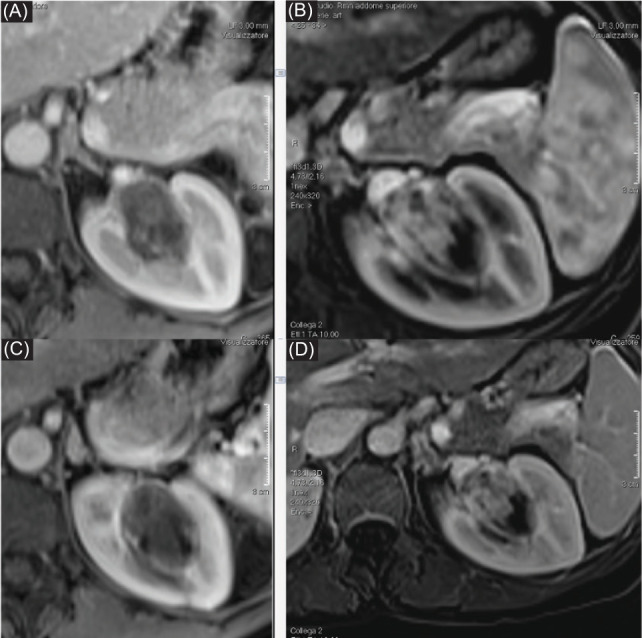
Two-year MRI control: (A, C) T1-weighted contrast-enhanced images, and (B, D) subtracted images demonstrated a reduced size of AML with only a small residual portion vascularized and without damage to healthy renal parenchyma.
